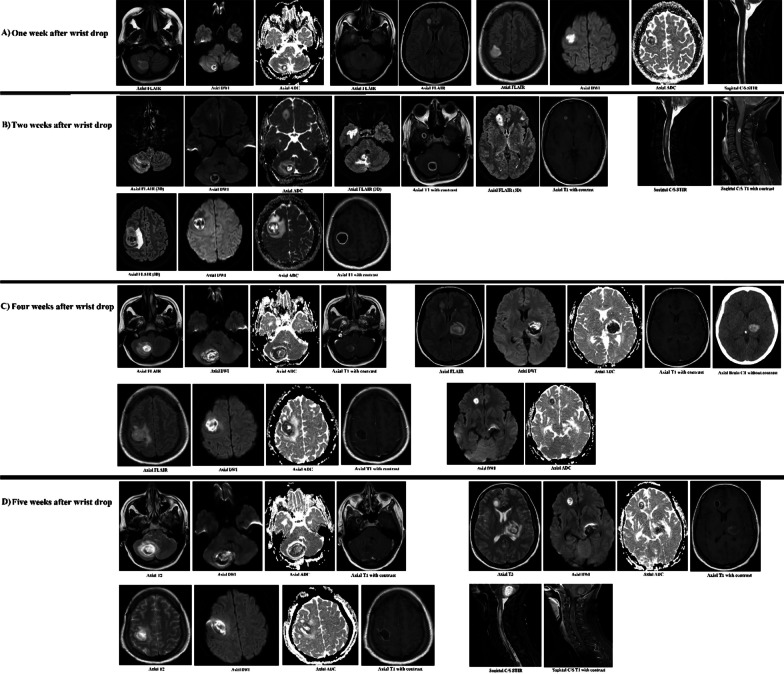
Brain magnetic resonance imaging (MRI) and brain computerized tomography (CT) of the patient with *Pseudomonas aeruginosa* brain abscesses (case 1). A Brain MRI without contrast performed 1 week after left upper limb weakness emergence (wrist drop) and before hospital admission shows multiple round brain lesions with high signal in T2-fluid attenuation inversion recovery (FLAIR) sequences and diffusion restriction in diffusion-weighted imaging (DWI) and apparent diffusion coefficient (ADC) sequences. The lesions in the right parietal and right cerebellum have the greatest sizes. In the last plane from the left, midsagittal short tau inversion recovery (STIR) MRI of the cervical spine (C/S), there is a longitudinal signal change in the cervical cord. B Brain MRI with contrast 2 weeks after wrist drop shows an enlargement of all the lesions shown in section A, all of them demonstrating a regular ring enhancement in contrast-enhanced-T1 MRI compatible with abscess. Note the considerable vasogenic edema surrounding the lesions as well as the round diffusion restriction of almost all the lesions in favor of pus collection. The longitudinal edema of the cervical cord is also enlarged in STIR with a small ring enhancement in the contrast-enhanced T1 MRI. C Four weeks after wrist drop and 2 weeks after admission the patient developed right hemiplegia. There is a round hyperdense hematoma in the left thalamus in brain CT corresponding with a high signal round lesion in FLAIR and low signals in DWI and ADC (blooming artifact in low B value) with peripheral vasogenic edema. If you look closely at section B, there is a very small hypersignal lesion with a faint ring enhancement in this location altogether in favor of hemorrhage in a small abscess in the left thalamus. All other abscesses are also increased in size compared with section B. D MRI performed 5 weeks after wrist drop, a few days before the patient’s death. All the abscesses are enlarged and there is a recollection of pus material in the right parietal and cervical cord abscess, which were drained surgically one week earlier
